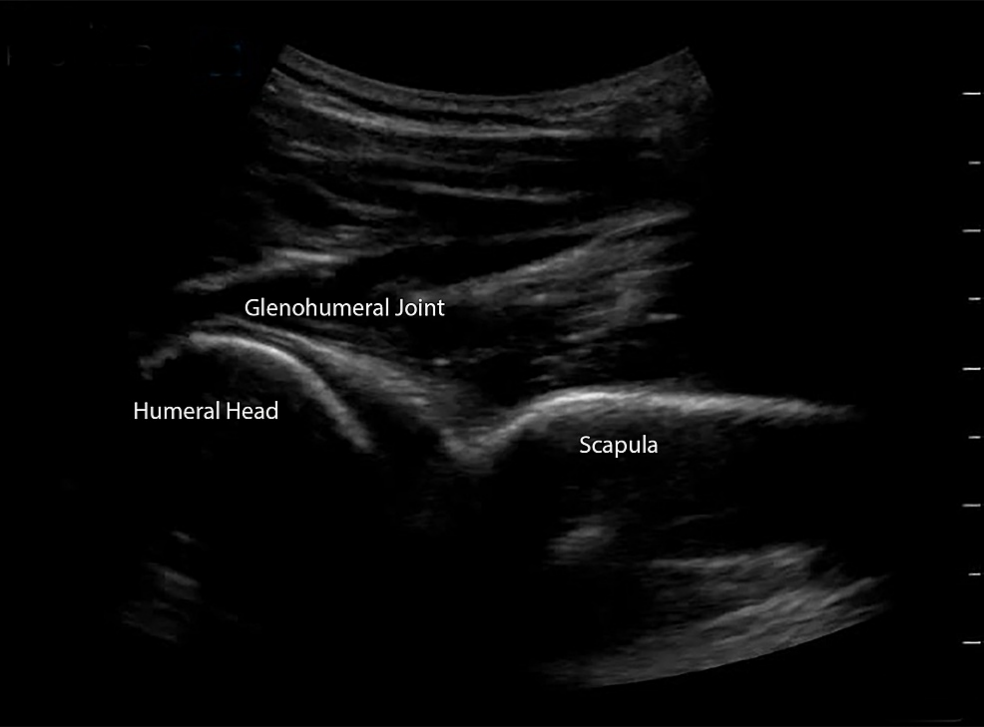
Glenohumeral Joint In this posterior view of the glenohumeral joint, the ultrasound probe is oriented transversely at the level of the scapular spine. A posterior view of the glenohumeral joint demonstrates proper horizontal alignment of the glenoid fossa of the scapula and the humeral head. The humeral head may be directly visualized moving within the glenoid fossa to confirm alignment. Lack of horizontal alignment of the scapula and the humeral head is pathologic and indicative of dislocation. The joint effusion may also be appreciated in this location.
